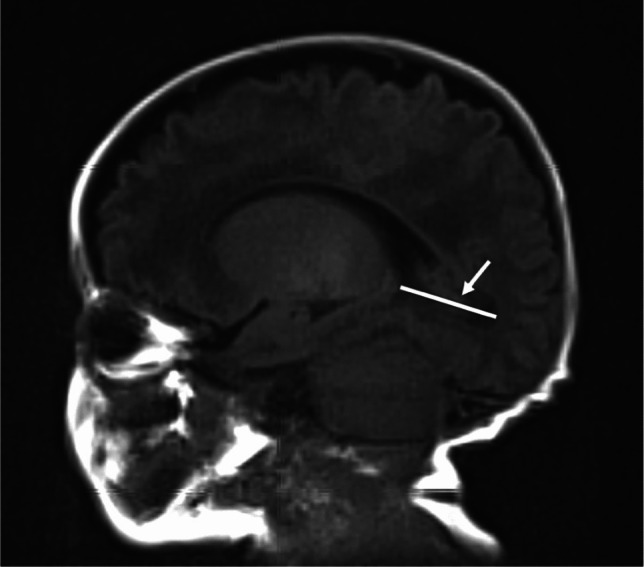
A 15-week-old boy born extremely premature at 26 gestational weeks with magnetic resonance imaging performed at term-equivalent age. Sagittal T1-weighted image without contrast shows thalamo-occipital distance on the right side (*arrow*). The infant had no intracranial haemorrhage or other pathology identified on cranial ultrasound in the neonatal period
